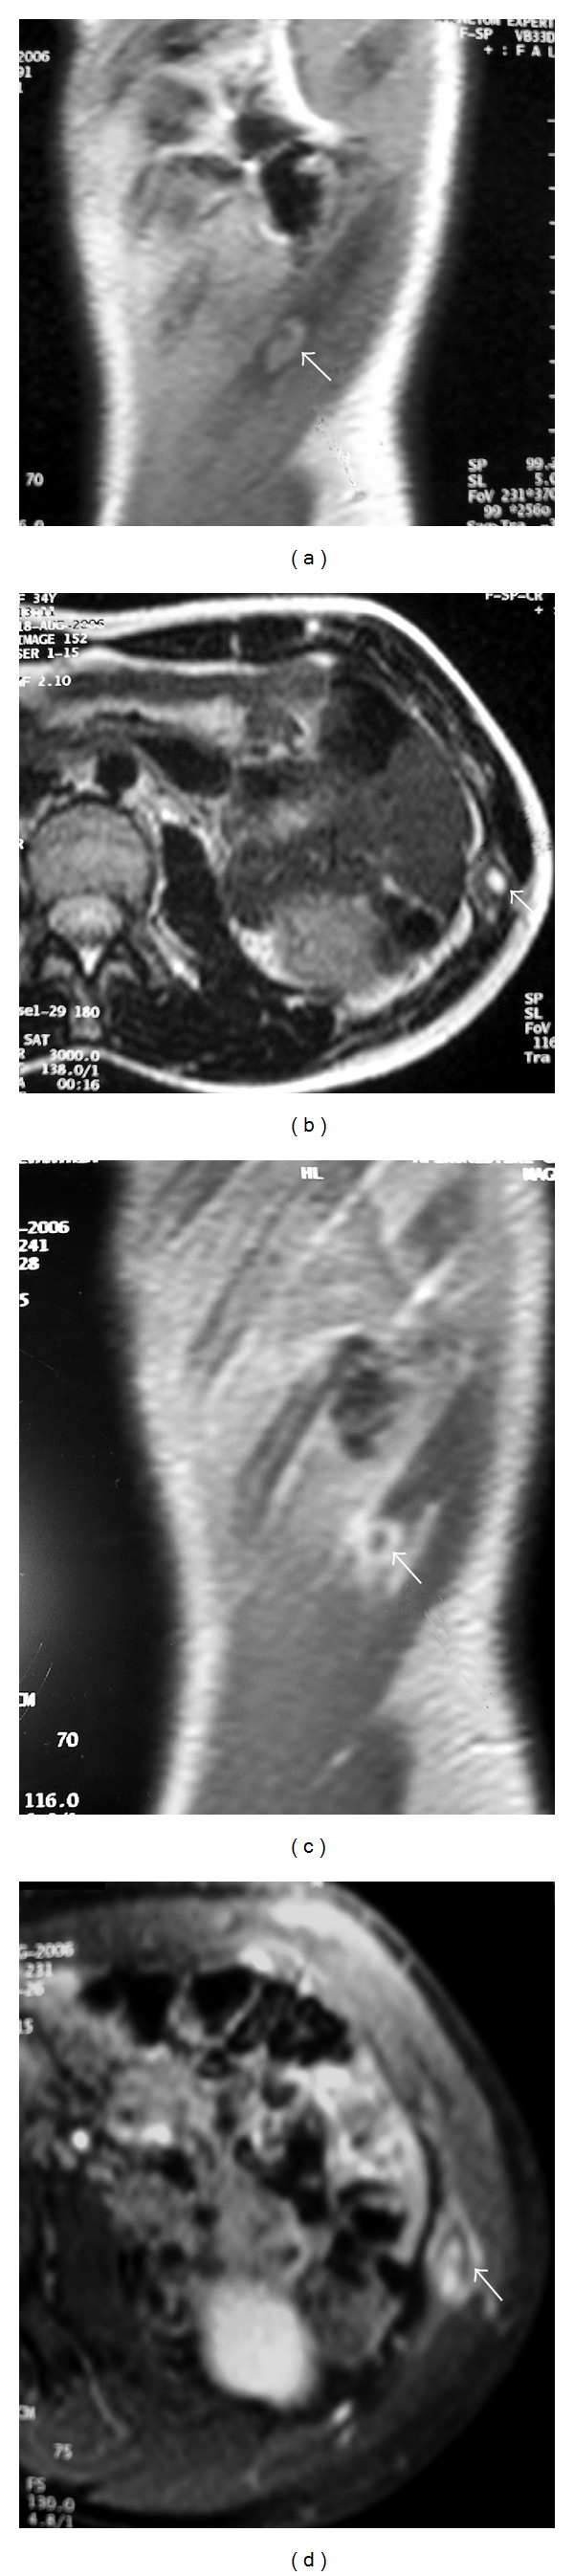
MR imaging of the tumor (arrows). (a) T1W, sagittal section shows an ovoid lesion with intermediate signal intensity, slightly higher than that of muscle. (b) T2W, axial section shows that the lesion has a high signal center and an intermediate signal periphery. After administration of paramagnetic contrast agent, there is both central and peripheral-periosteal enhancement. ((c) sagittal T1W + CM, (d) axial T1W FatSat + CM). The perilesional soft tissues show no signs of invasion. The findings are atypical.
